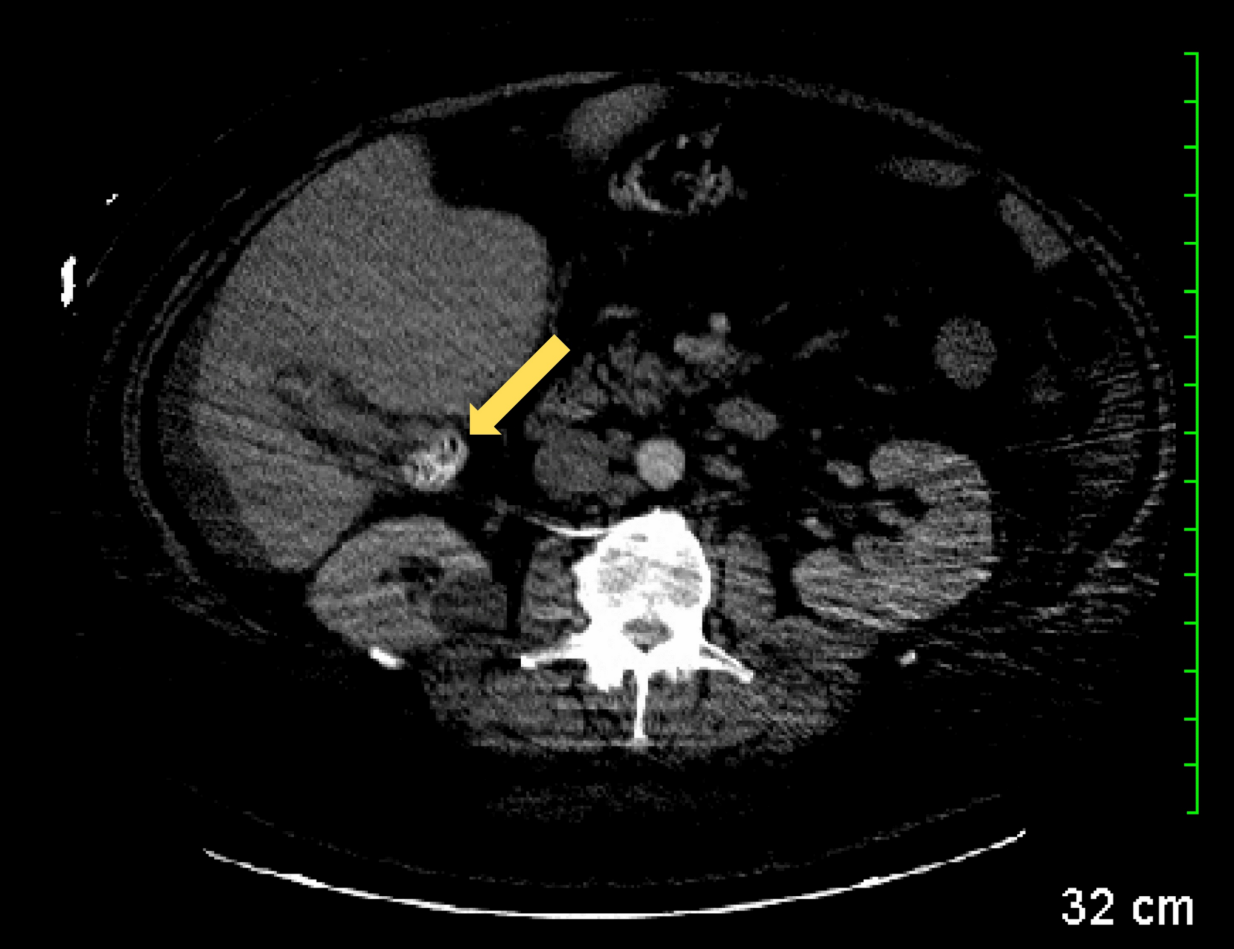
CT scan revealing a partially collapsed gallbladder with small stones inside (yellow arrow). CT: computed tomography
